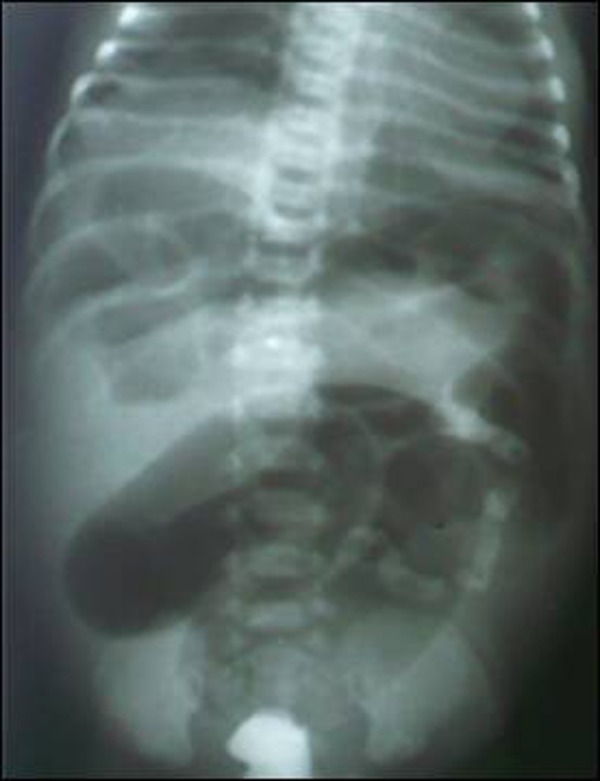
Figure 1: X-ray abdomen erect with gastrografin enema, showing small caliber of colon and filling defects
